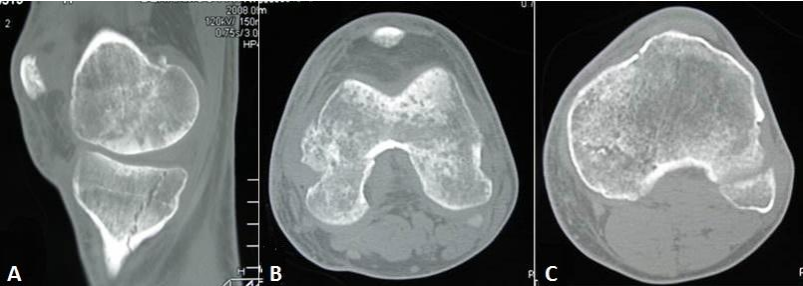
Control CT scan of left knee showing a perfect consolidation of bone lesions. (A) sagittal; (B) axial femoral condyle; (C) axial tibia plateau
